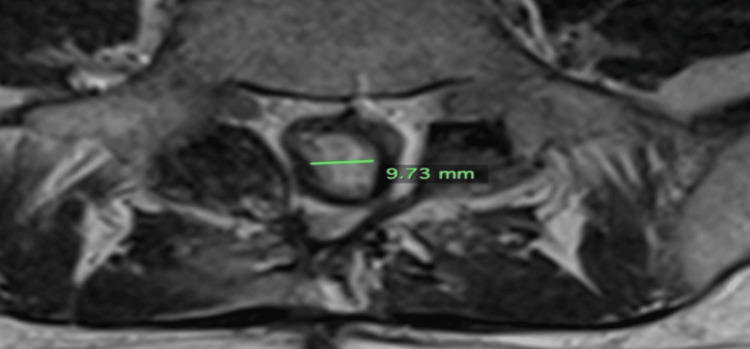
Axial image from an MRI L-spine with contrast obtained approximately two months post-cryoablation dated Aug 1, 2023. The tumor measures 0.97 cm transversely.
